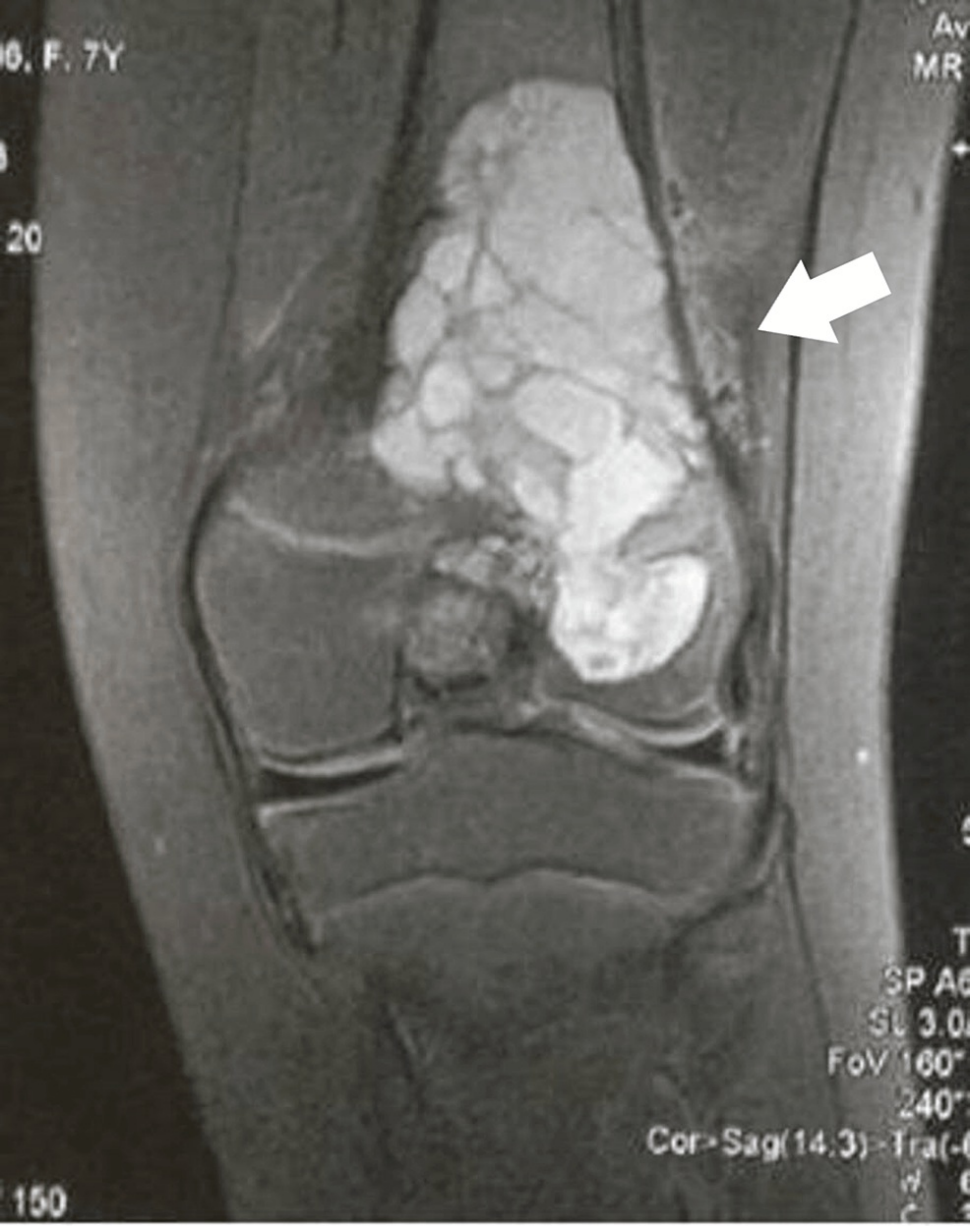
A coronal T1-weighted MRI image showing multiple fluid-fluid levels in the metaphysis, crossing the physis, and extending into the epiphysis.
